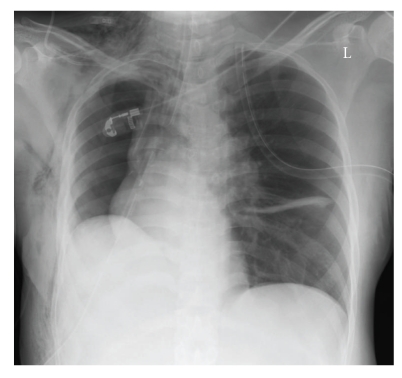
Chest X-rays of the patient after insertion of a chest tube and re-expansion of the left lung.
